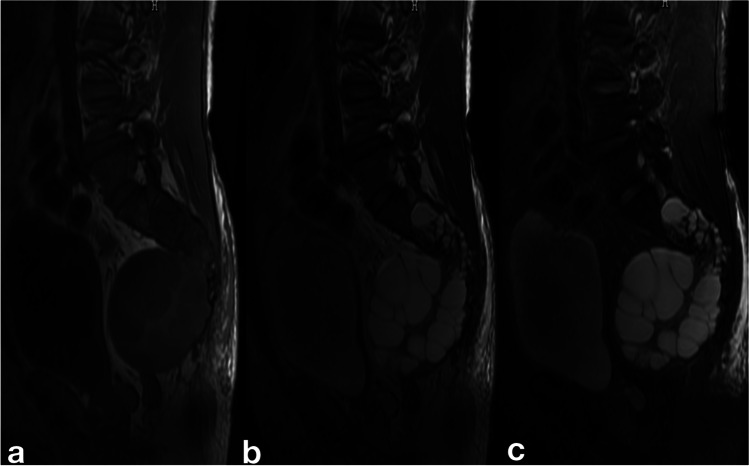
a–c Sagittal MR imaging demonstrated a polycystic round lesion with low signal intensity on T1WI (a), high signal intensity on T2WI (b), and high signal intensity on STIR (c). There was linear low signal on T1WI, T2WI, and STIR in the septa and cyst’s wall. The damaged vertebrae ranged from the 2nd to the 5th sacral vertebra
